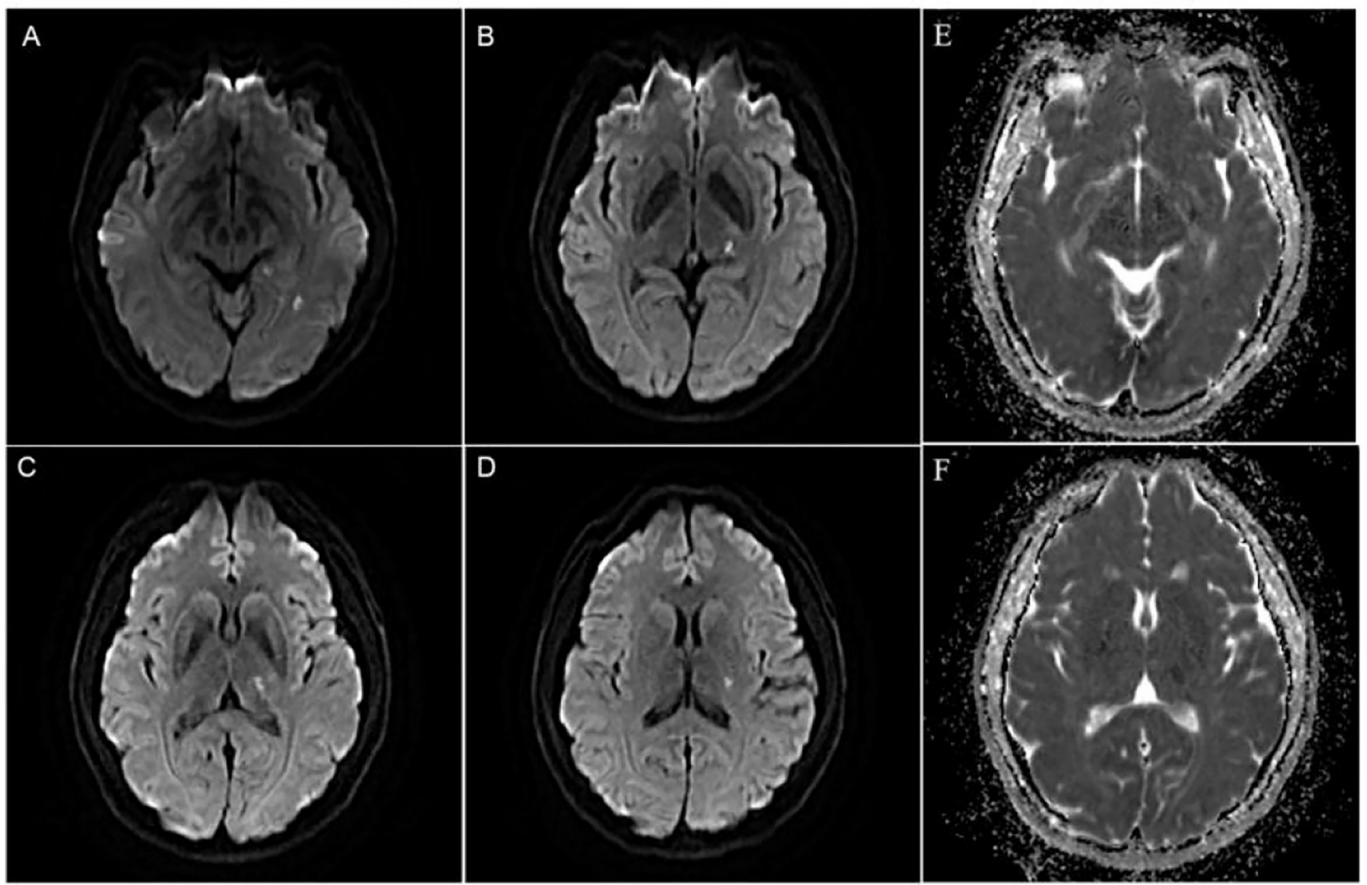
(A–D) Diffusion weighted imaging(DWI) showed multiple and small areas of acute infarction on the left basal ganglia, thalamus, and temporal lobe. (E,F) ADC image showed multiple and small areas of low signal areas on the left basal ganglia, thalamus, and temporal lobe.
